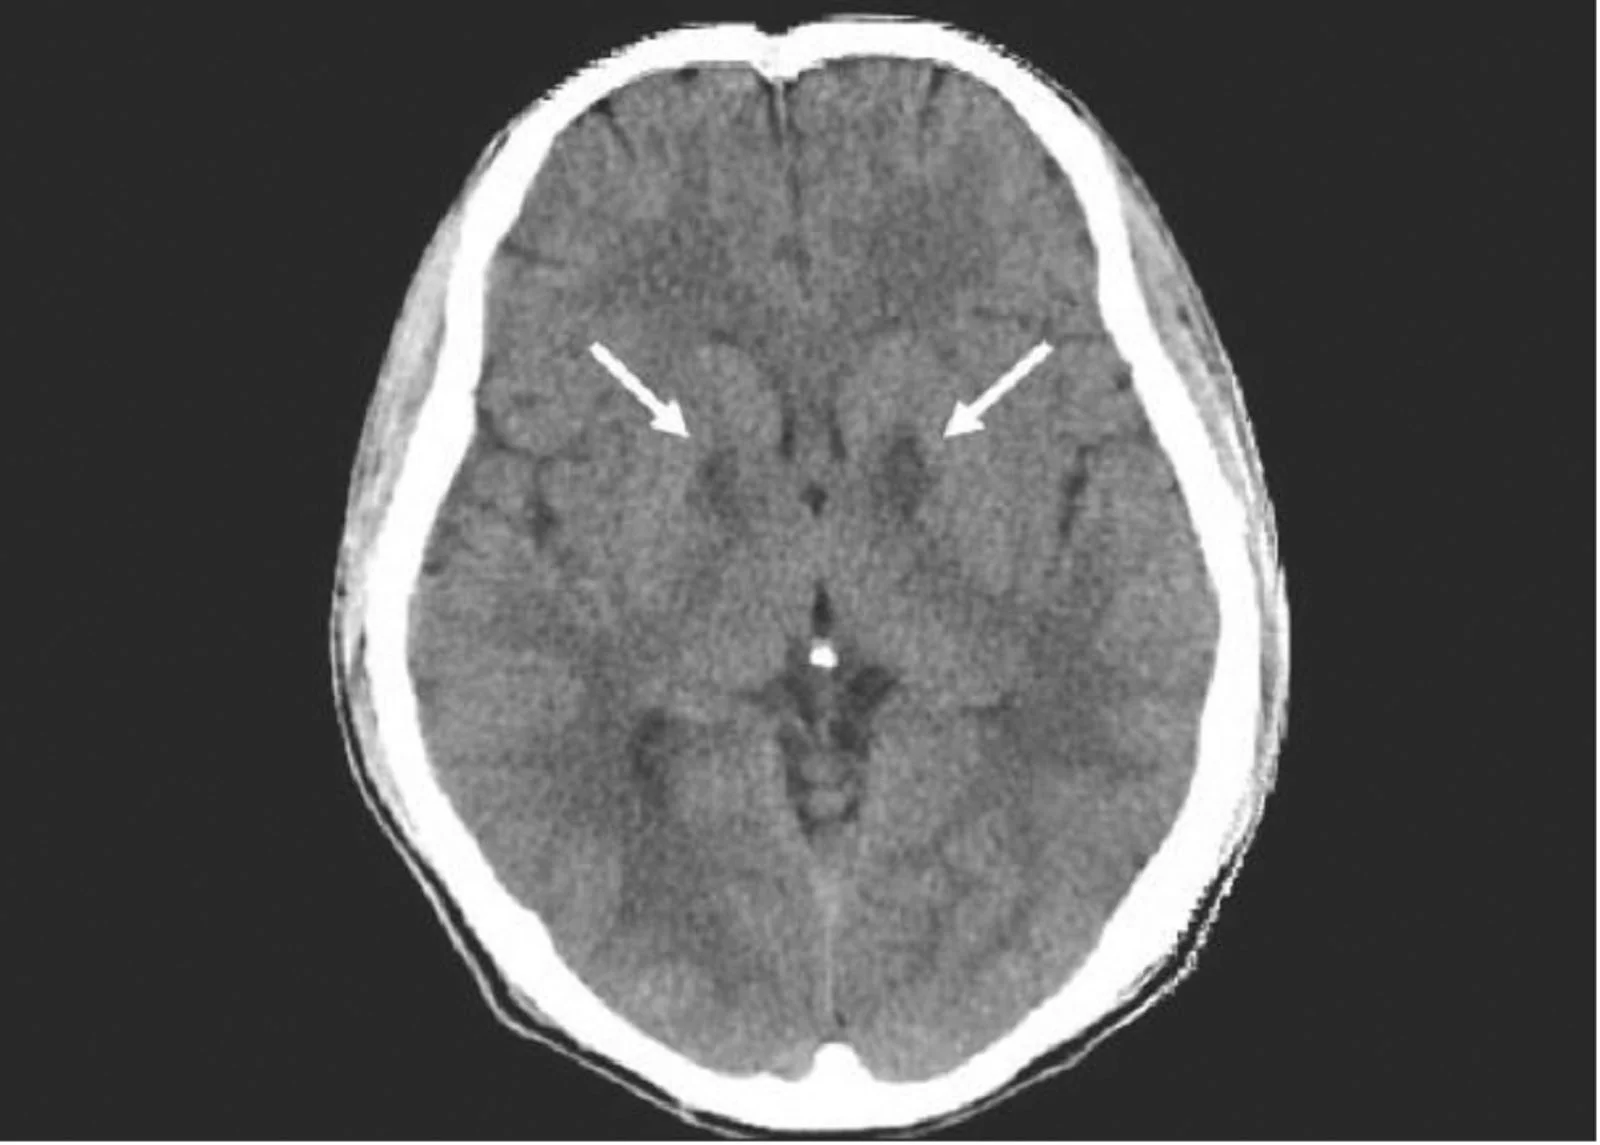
CT performed at the time of admission demonstrated hypodense lesions within the bilateral globus pallidus. CT = computed tomography.
